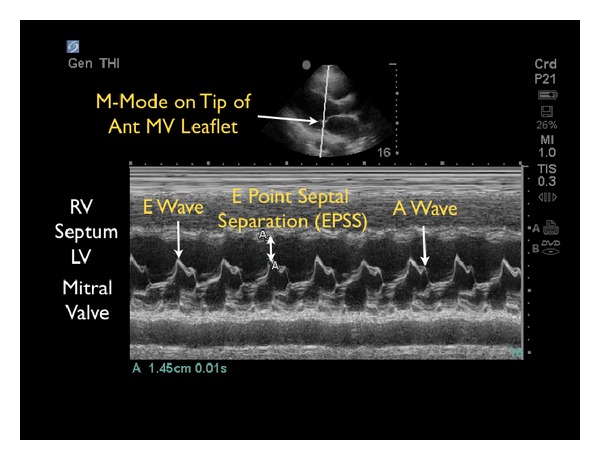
E-point septal separation with decreased contractility. M-mode Doppler tracing. RV: right ventricle, LV: left ventricle.
